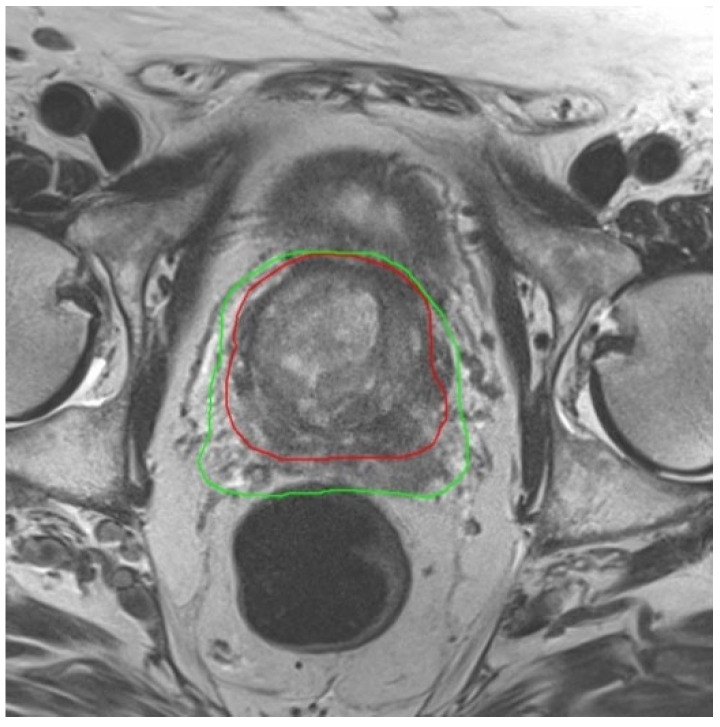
Image of MRI 1 acquired before beginning of ADT with the 60 Gy Isodose-line (green) in treatment plan. The red line is the 60 Gy Isodose-line from treatment plan based on MRI 2 after shrinkage of the prostate by ADT. The dose burden of the rectum is clearly lowered by using a new MRI after ADT.
